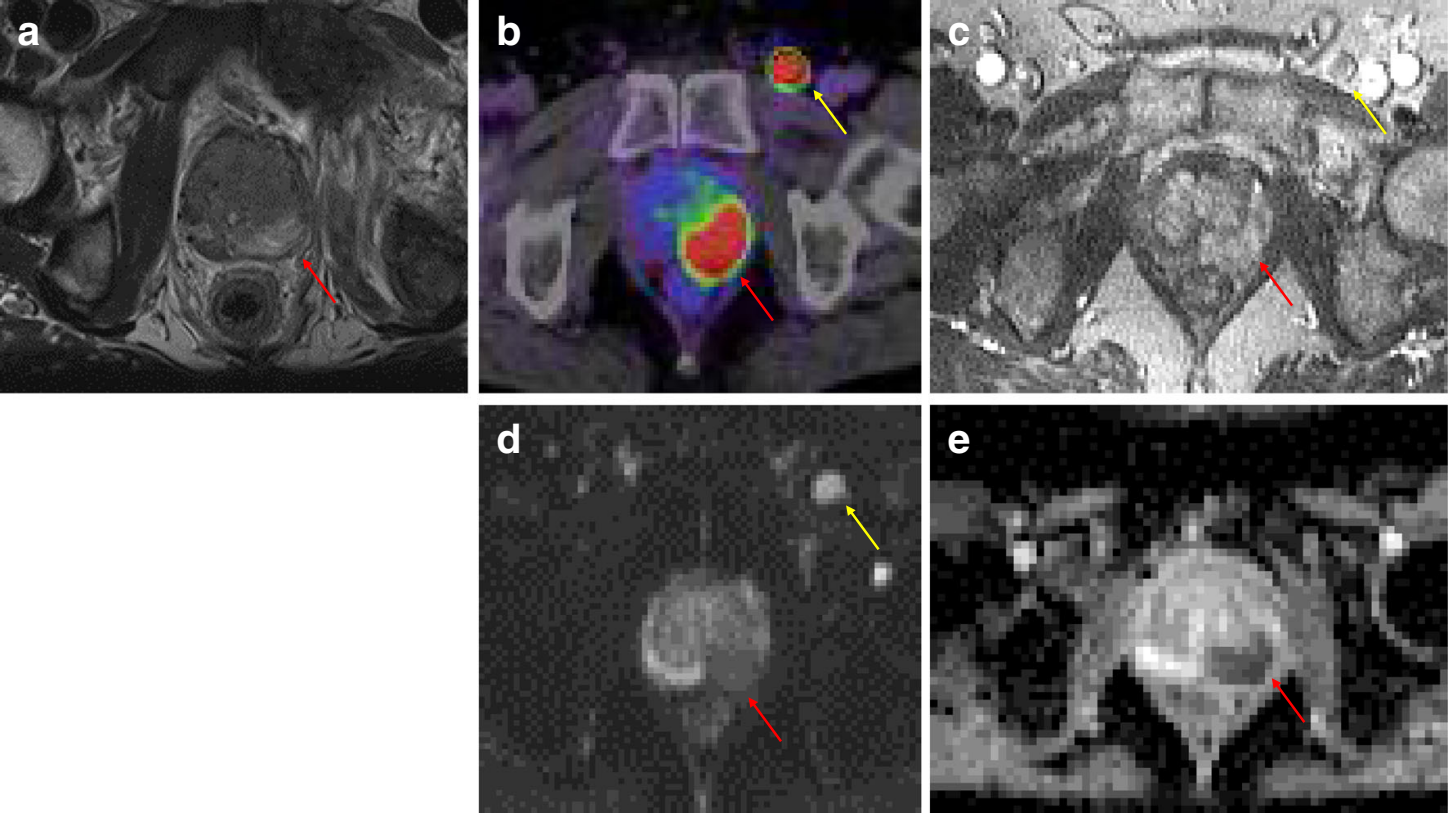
A 61-year-old patient with a biopsy-proven prostate cancer in the left peripheral zone. According to MP-MRI (a, T2w; c, CE-T1w; d, DWI, e, ADC), the tumor affected PI-RADS segments 9Pand 10P. In PSMA-PET (b, *red arrow*), a near-total agreement (tumor affecting PI-RADS segments 9P, 10P and 10A) was scored. PSMA-PET also diagnosed a loco-regional lymph node metastasis (b, *yellow arrow*) that was retrospectively also delineable in CE-T1w (c), DWI (d) and ADC (e)
